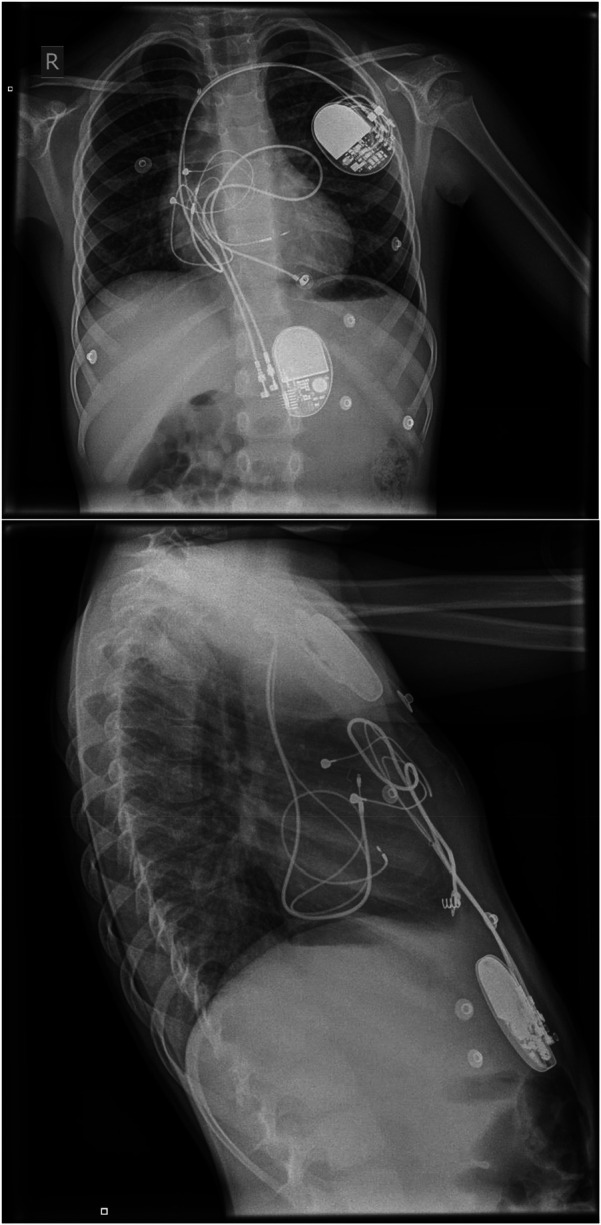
Posteroanterior (PA) and lateral x-ray chest showing a dual chamber pacemaker with its wire terminating in the right atrium and right ventricular septum. Another infradiaphragmatic dual chamber pacemaker with epicardial leads is also noted.
